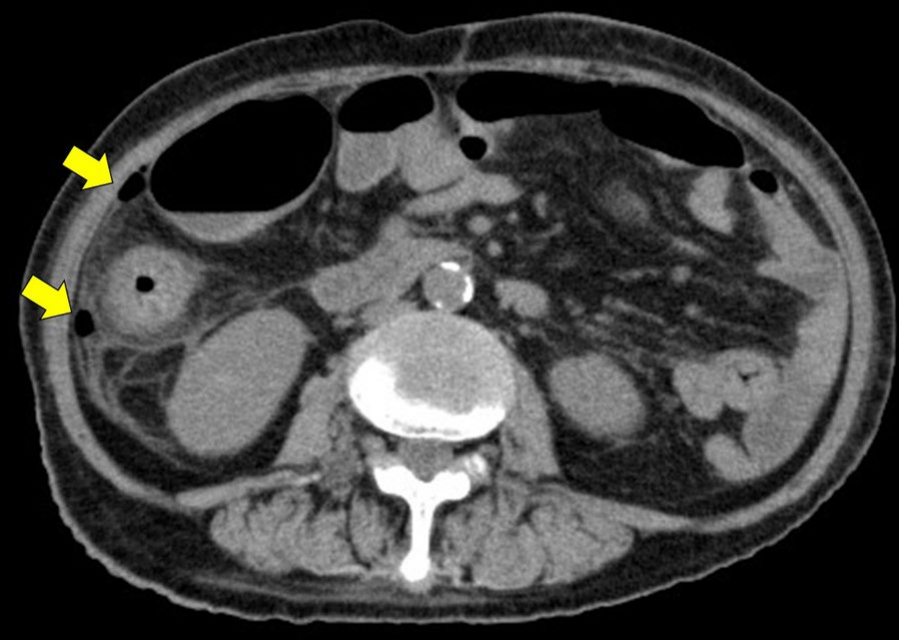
Abdominal CT of Case 1. Wall thickening and free air around the ascending colon are seen. Perforation of the ascending colon was suspected
